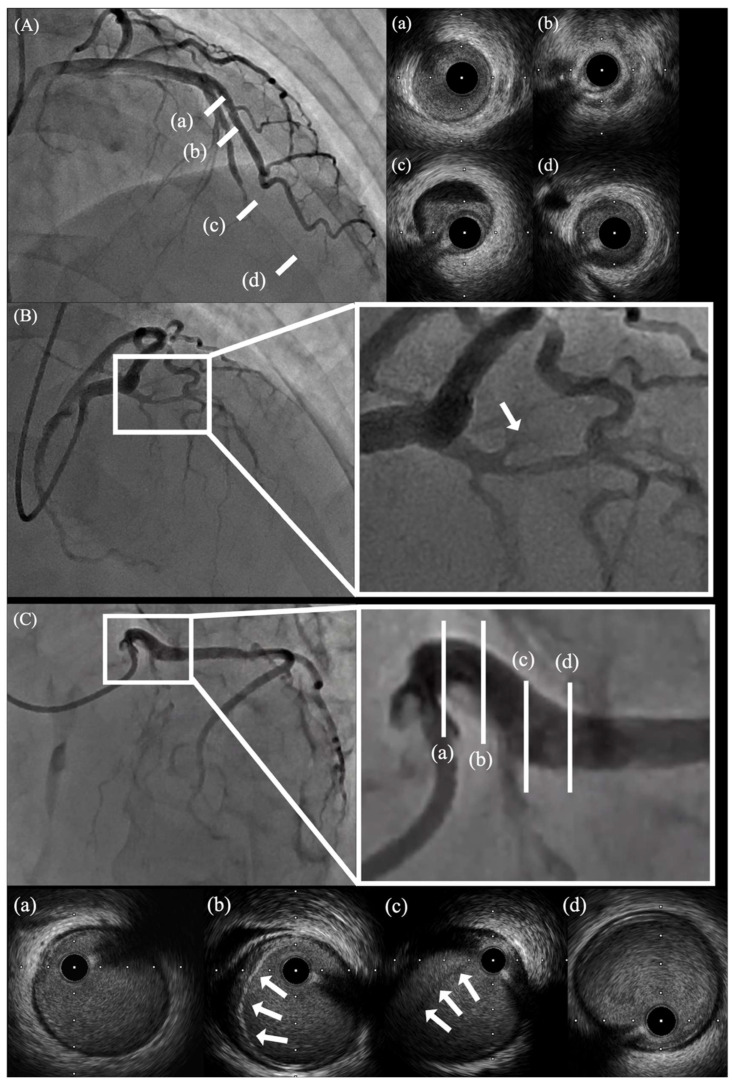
Intracoronary imaging: IVUS. (A) IVUS findings of the LAD in a case of type 4 SCAD. (a) No evidence of dissection proximal to the first septal branch. (b,c) Luminal narrowing caused by intramural hematoma. (d) Distal segment without extension of the dissection. (B,C) IVUS findings from the same case demonstrating type 4 SCAD extending from the proximal LAD. (B) The arrow indicates the site of occlusion. (C) IVUS images obtained from the LCX toward the LMT. (a) No abnormal findings in the mid-LMT. (b) The distal LMT represents the entry point of the dissection (arrow), showing a false lumen separated by an intimal flap. (c) At the bifurcation from the LMT into the LAD and LCX, the left side of the image corresponds to the LAD, demonstrating a false lumen and intramural hematoma separated by a flap (arrow), resulting in compression and narrowing of the true lumen of the LAD. (d) No extension of the dissection into the LCX. Abbreviations: IVUS = intravascular ultrasound; LMT = left main trunk.
